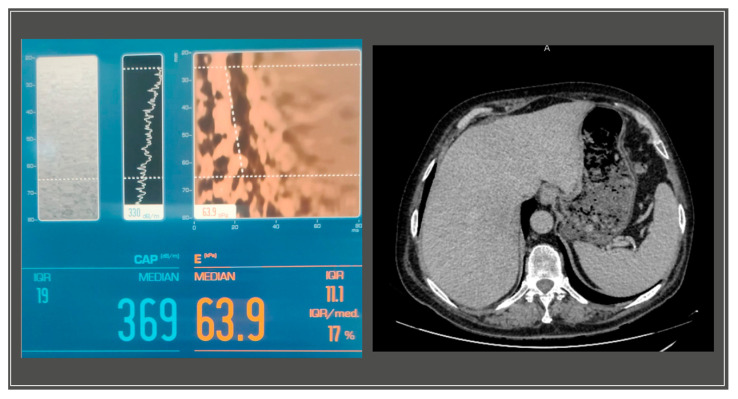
Liver imaging with S3F4 score on Fibroscan® and abdominal CT with mild liver enlargement.
